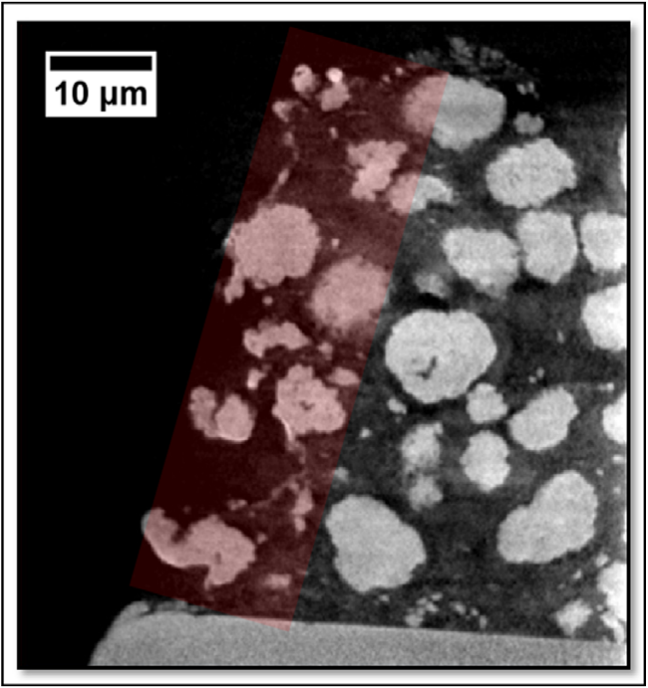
Vertical plane radiograph of the side of Li‐NMC electrode pillar, laser‐affected zone (LAZ) highlighted in red.
